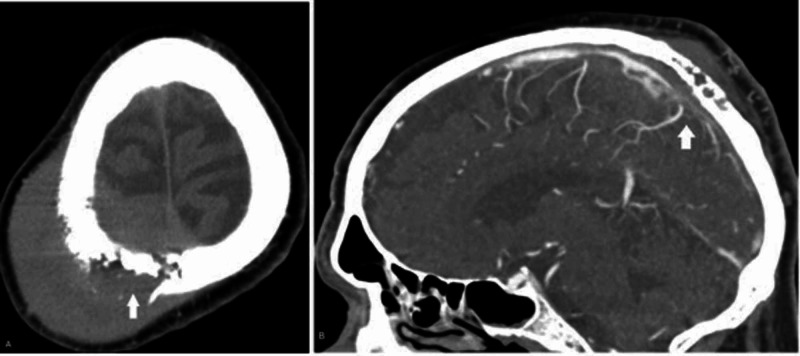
A. Axial CT head demonstrating a large subcutaneous mass with infiltration of the cranial vault and bony destruction. B. Sagittal CTA demonstrating bony destruction and superior sagittal sinus involvement. CT: computed tomography; CTA: CT angiography
